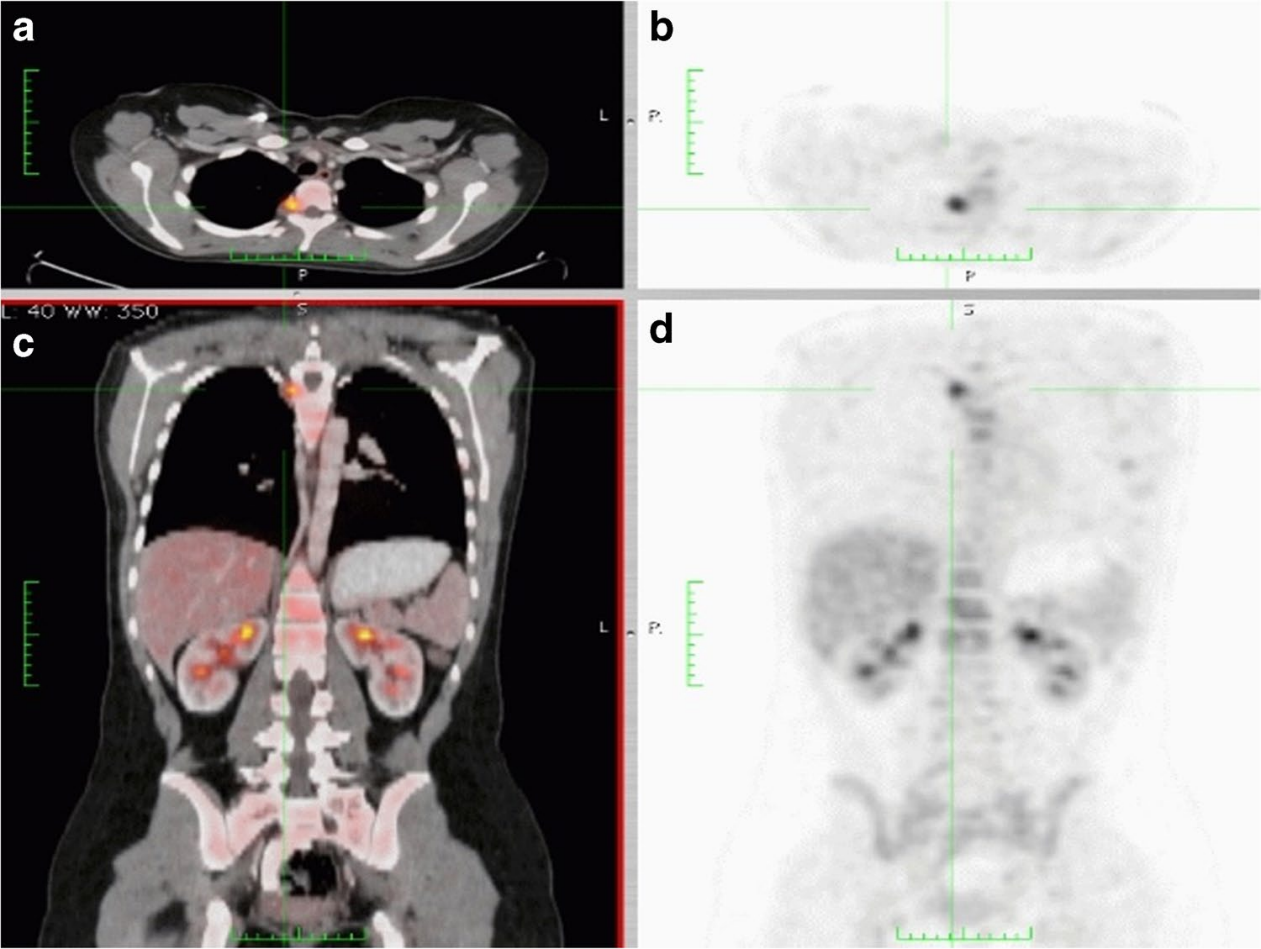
Axial (a, b) and coronal (c, d) fluorodeoxyglucose-positron emission tomograhy-computed tomography scans of a 15-year-old girl (patient 2) just before completion of treatment of recurrent rhabdomyosarcoma show new osseous metastases in the spine and pelvis
